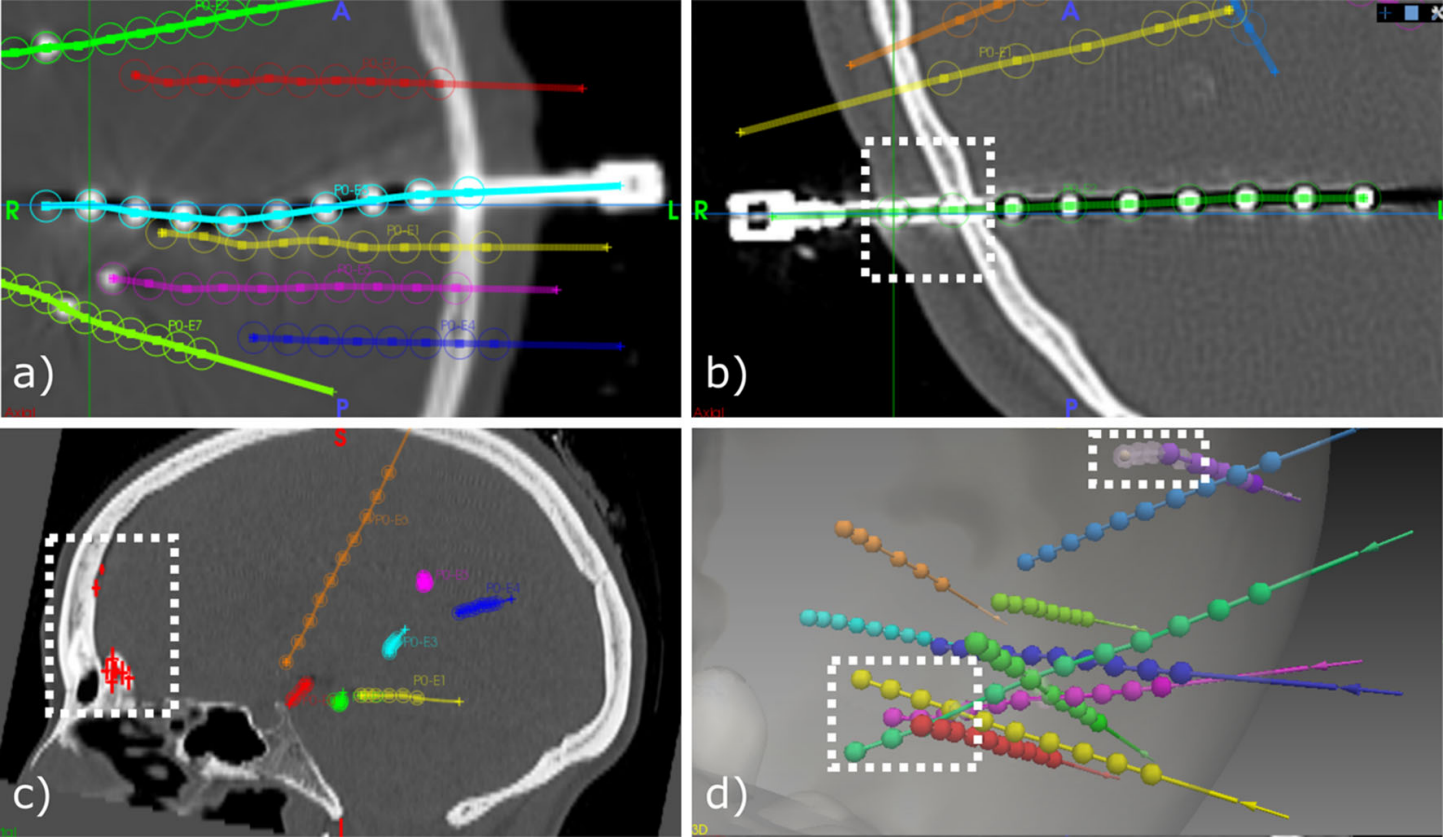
Examples: a segmented bolt head and contacts of electrodes overlaying CT; b contacts predicted at the skull and scalp level; c 22 FP (red marks along the skull); and d our worst case with 3 contacts not segmented due to crossings and 4 FNs
